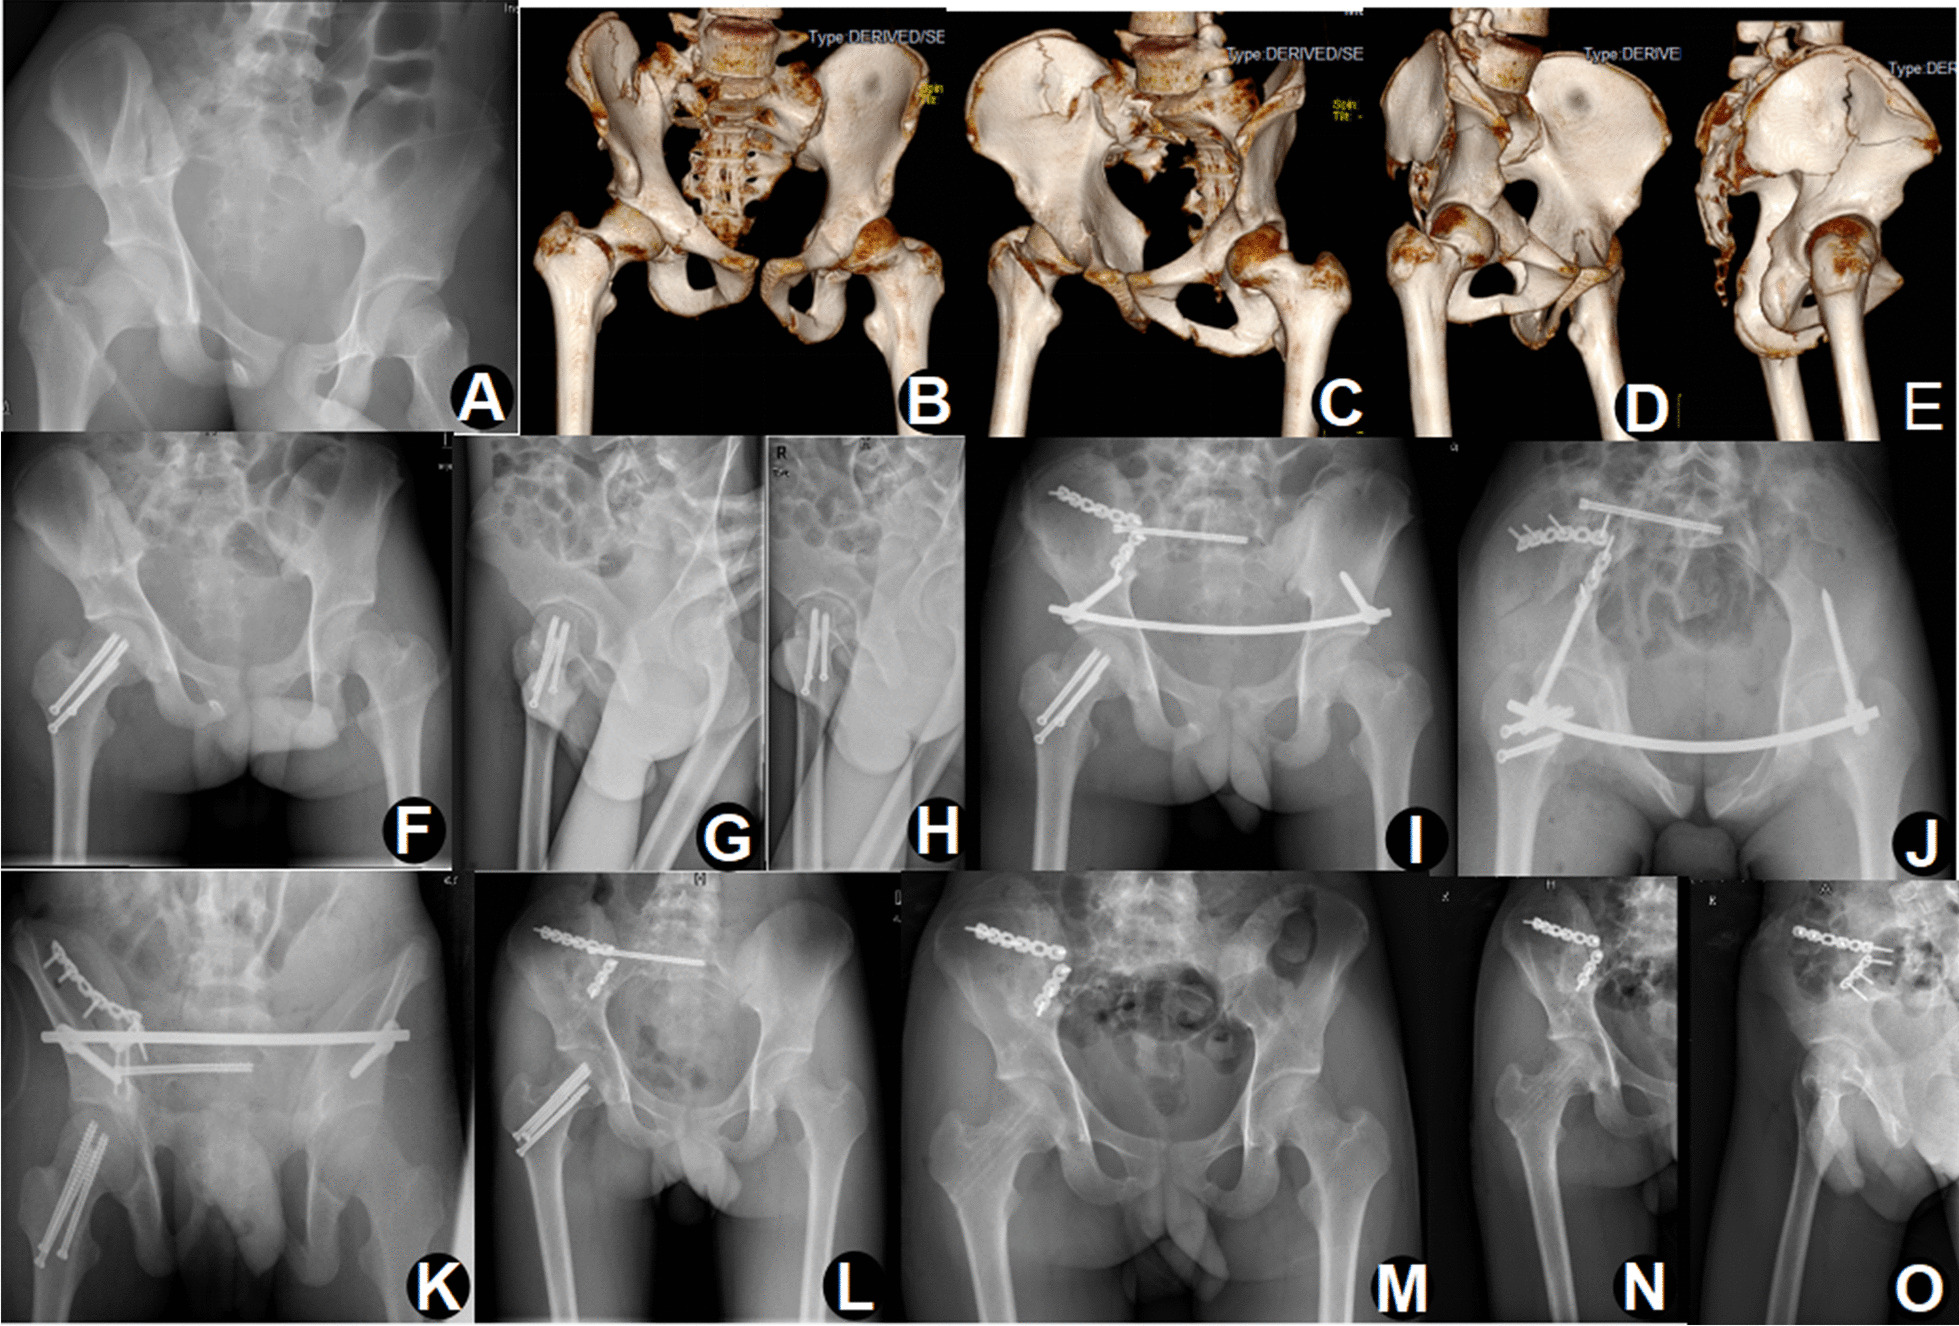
A 16-year-old male treated with damage control orthopaedics. Initial radiographs (A) and three-dimensional computed tomography images (B–E) showing a type C floating hip. Closed reduction and internal fixation of femoral neck fracture on the third day after admission (F–H). Percutaneous sacroiliac screw fixation of the posterior ring, INFIX fixation of the anterior ring, and anterior fixation of the acetabular fracture by the lateral window of the IL approach on the tenth day (I–K). The removal of INFIX 1 year after surgery (L). The removal of cannulated screws and sacroiliac screw 1.5 years after surgery (M–O)
